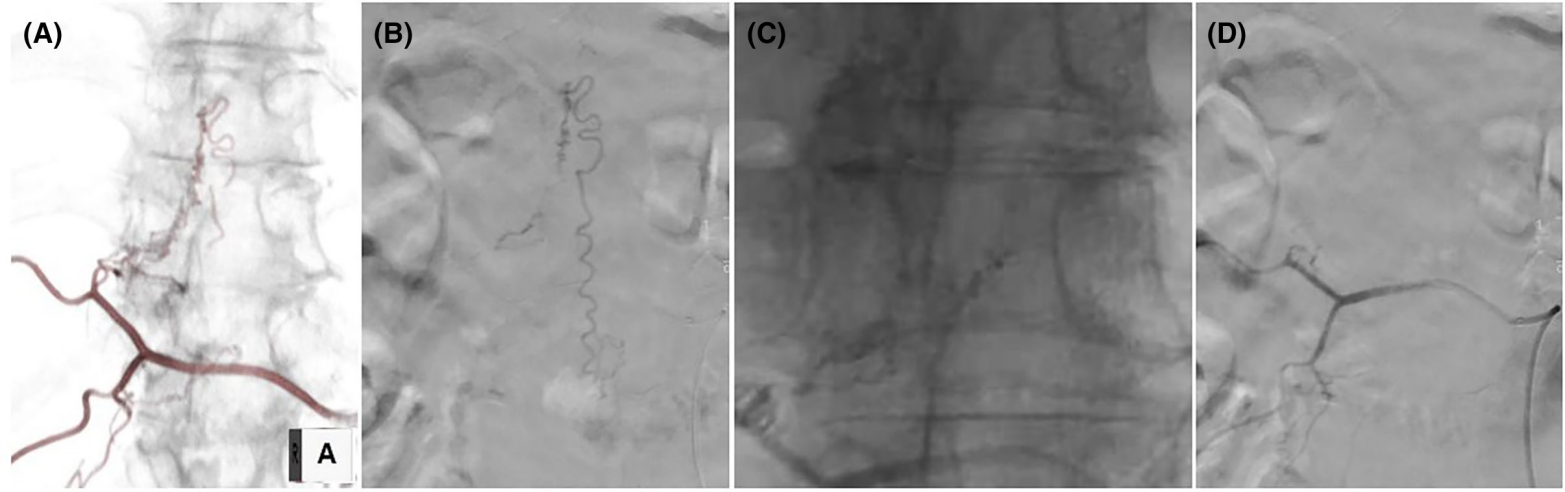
(A) Three‐dimensional digital subtraction angiography (DSA) image of the right Th11 and Th12 segmental arteries showing the spinal dural arteriovenous fistula (SDAVF), intradural arterialized vein, and dilated premedullary veins. (B) Anteroposterior (AP) view DSA image of the right Th11 segmental artery showing a shunt lesion from selective catheterization of the right Th11 radicular branch with a tangle of arterialized veins noted in the central spinal canal. The fistula is noted at the right Th11 nerve sheath. (C) Post‐treatment radiograph showing the presence of n‐butyl‐2‐cyanoacrylate. (D) Post‐treatment AP view DSA image of the right Th11 and Th12 segmental arteries showing the disappearance of SDAVF.
